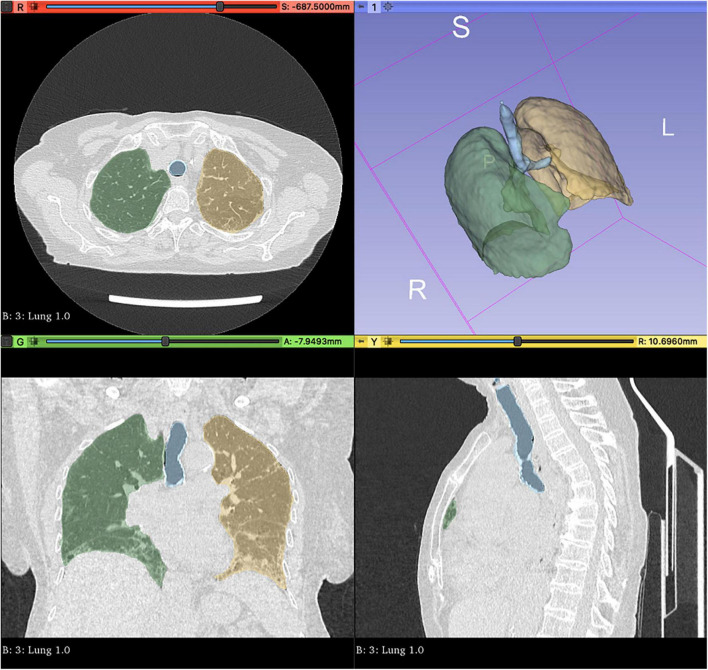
Automated whole lungs and trachea segmentation using 3D Slicer (http://www.slicer.org) *via* the Lung CT Analyzer project (https://github.com/rbumm/SlicerLungCTAnalyzer/).
